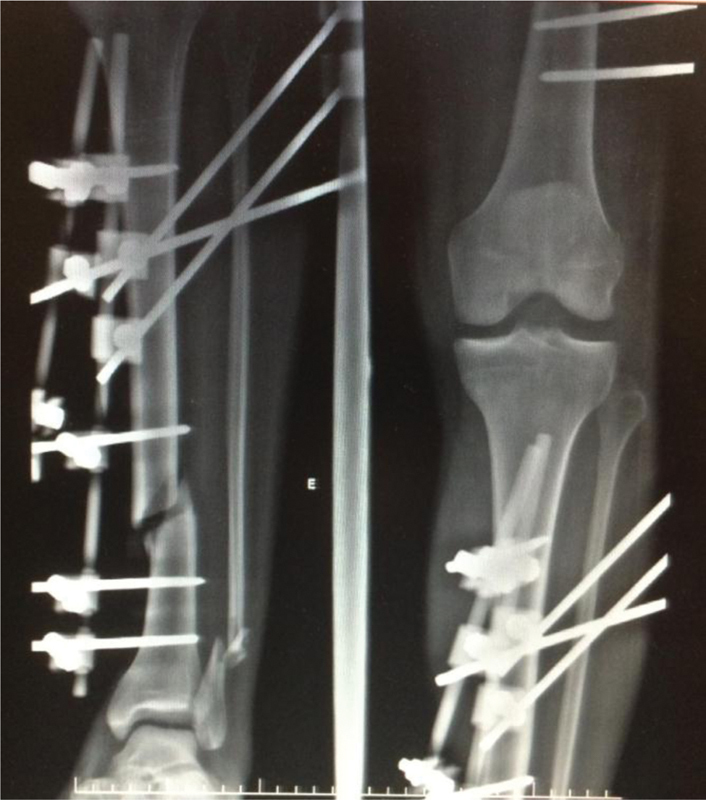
Plain radiograph of the left leg with the bridging external fixator applied to the tibial fracture and dislocated knee. Note the opening of the lateral compartment of the knee, suggesting PLC lesion. PLC, posterolateral corner.
